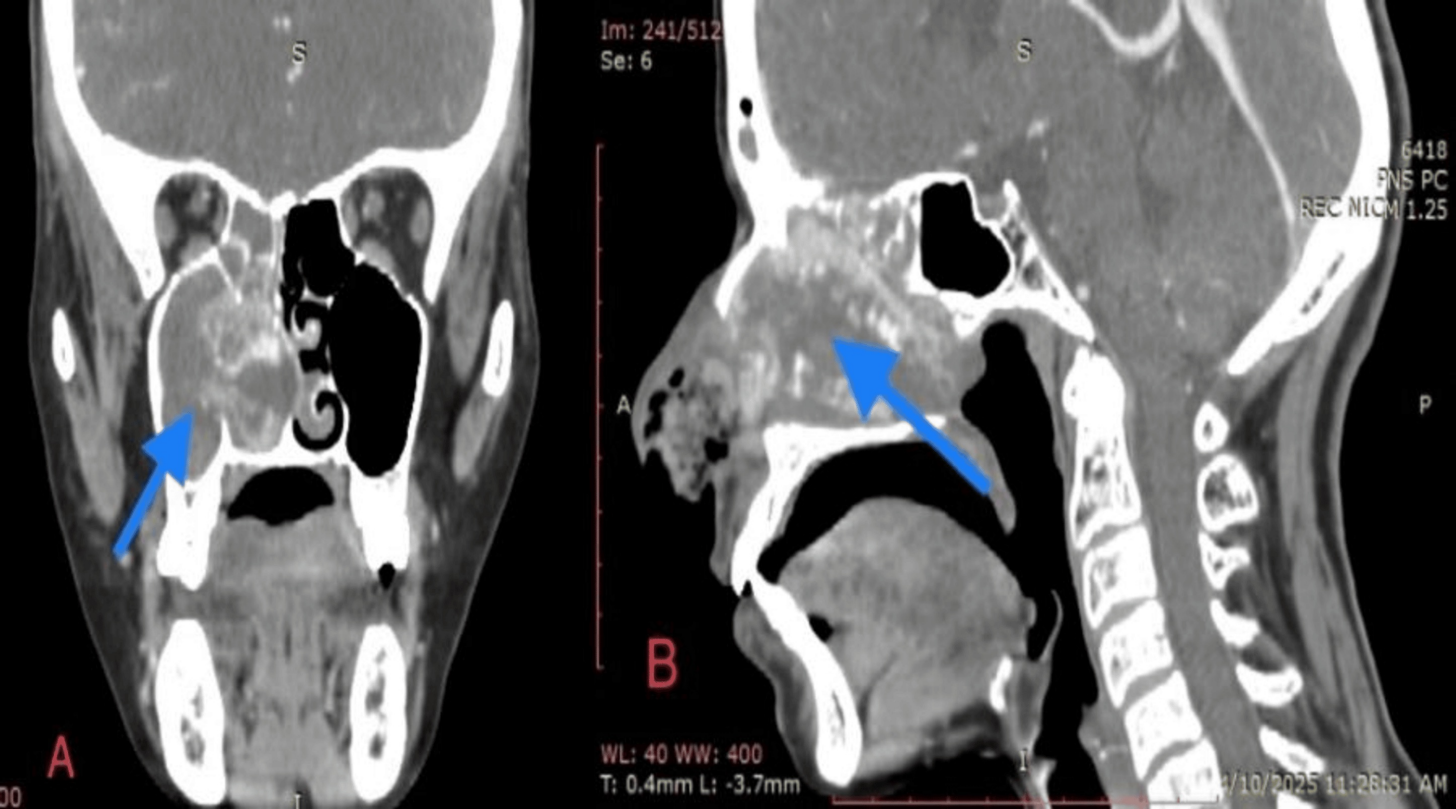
Coronal (A) and sagittal (B) planes of a contrast-enhanced CT scan of the nose and paranasal sinuses A contrast-enhanced CT scan of the nose and paranasal sinuses revealed enhancing polypoidal mucosal thickening and total tissue opacification of the right frontal, ethmoidal, maxillary, and sphenoid sinuses, with the epicenter at the right maxillary antrum. It was associated with widening of the osteomeatal complex and appeared to merge with the right nasal turbinates, almost totally obliterating the right nasal cavity. This resulted in remodeling and expansion of the sinonasal cavity, producing contralateral mass effect and extending up to the posterior choanae. Multifocal rarification and erosion of the medial wall of the maxillary sinus with erosion of the intervening bony septa were noted. No definite intraorbital or intracranial extension was seen.
